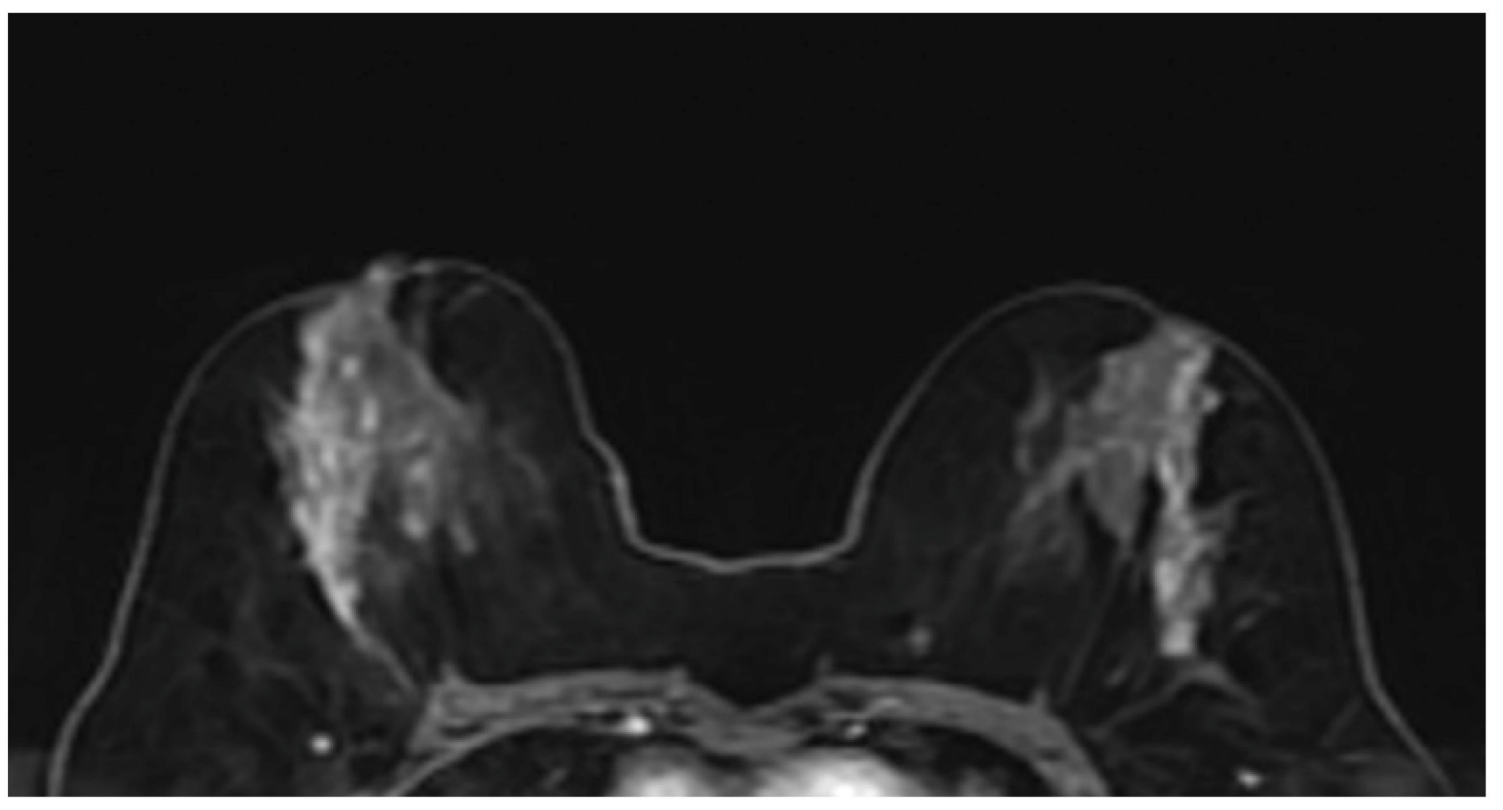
MRI image showed segmental heterogeneous enhancing NME lesion on the right and linear heterogeneous enhancing NME lesion on the left in a 46-year-old patient. Right breast biopsy result: DCIS, left breast biopsy result: atypical ductal hyperplasia.
